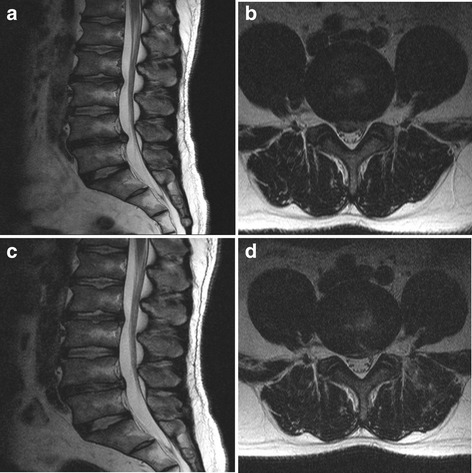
MR images showing broad based central disc extrusion at the L4–5 (a and b). After the PELD, MR images showing sound decompression (c and d)
